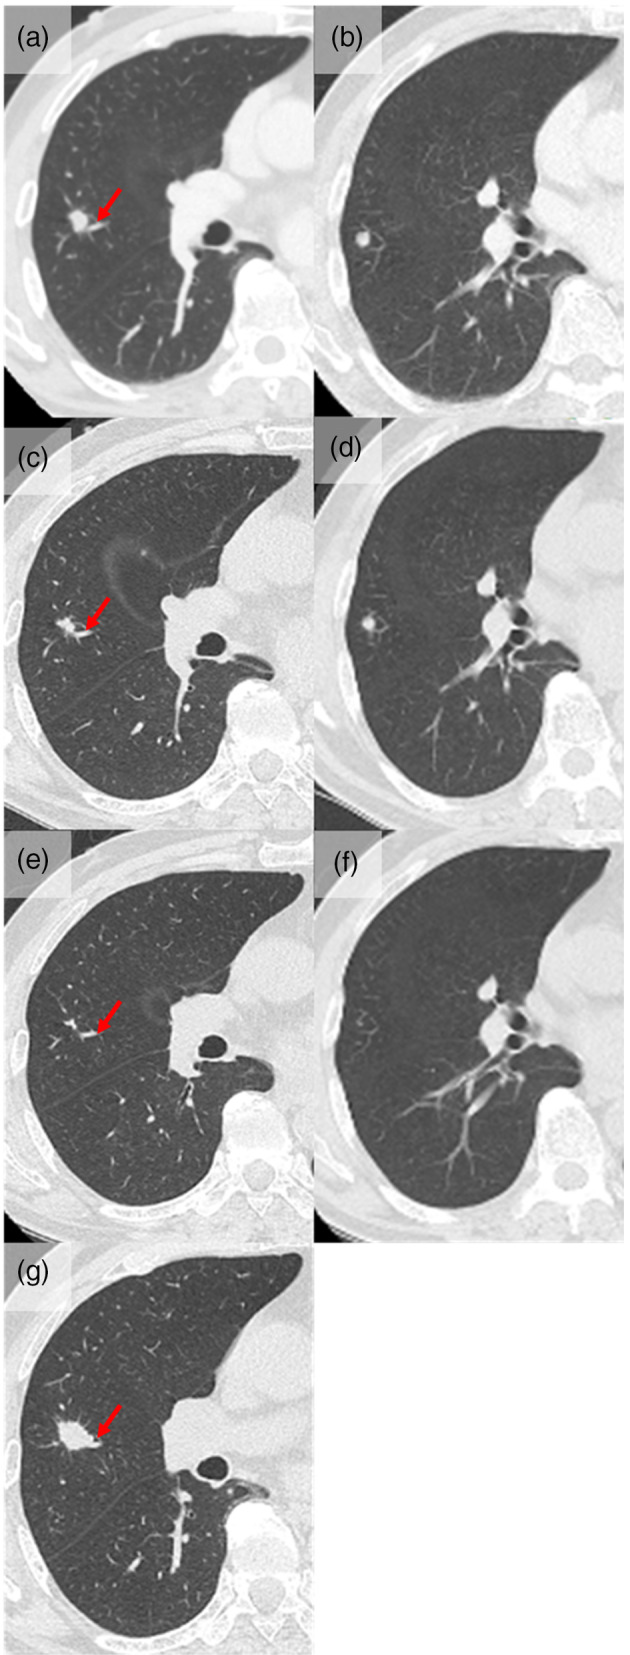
Chest computed tomography (CT) of the tumor. (a) CT revealed a 10.5 x 9.7 mm nodule located in the peripheral section of B2b, close to V3aii (red arrow), and (b) a 7.8 x 5.4 mm nodule located in S2 at the first visit. (c) One month later, the larger nodule had shrunk to 8.1 x 8.4 mm. (d) The size of another nodule had not changed. (e, f) Four months later, both nodules had shrunk to an almost unrecognizable size. (a, g) Two years later, CT revealed a 19.3 x 10.4 mm nodule located in the peripheral section of B2b, close to V3aii (red arrow), in exactly the same place as the larger nodule revealed 2 years previously.
